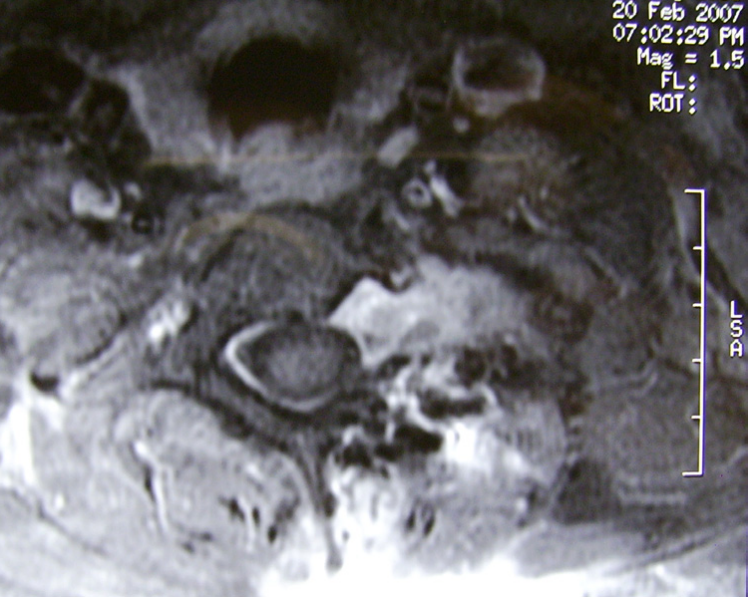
Transverse section of T2 weighted MRI demonstrating complete removal of the neuroma from the left T1-2 intervertebral foramen and the spinal canal.
